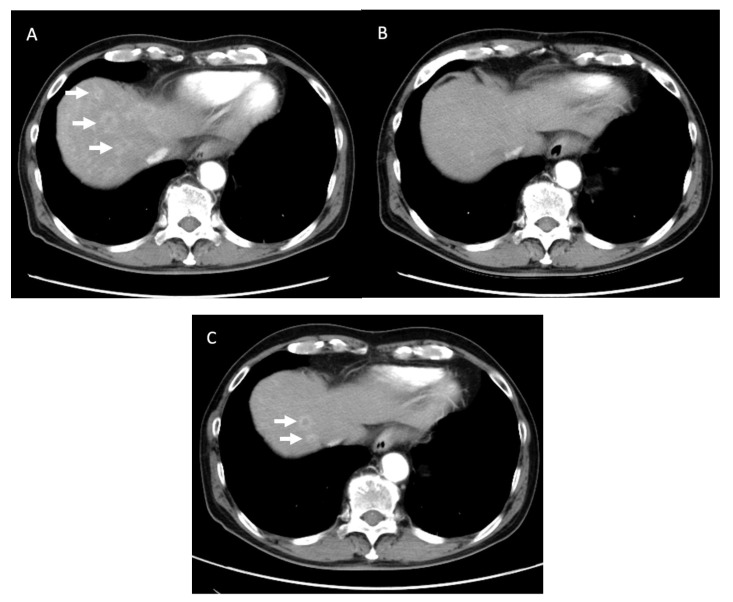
Computed tomography (CT) findings of Patient #1. (A): CT showed multiple metastatic lesions (white arrow) in the liver before chemotherapy. (B): All liver metastasis disappeared on CT after chemotherapy. (C): Cancer recurrence in the liver (white arrow) was confirmed 8.5 months after conversion surgery.
